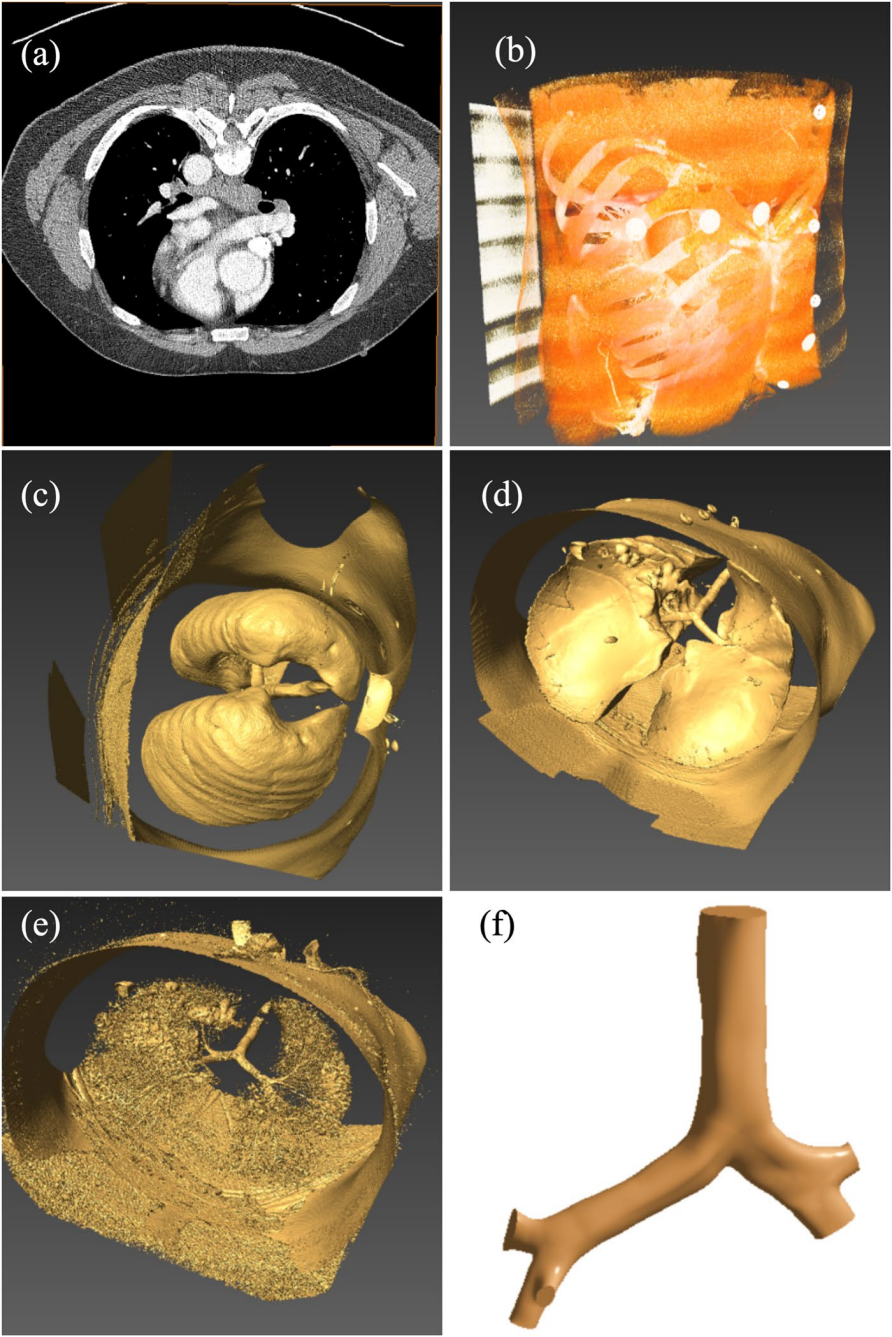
Geometry generation from the CT-based DiCom images of a healthy adult, (a) visualization of the raw DiCom images, (b) airway tree with skeleton, (c) 3D lung airway with right and left lobe, (d) bifurcation views with left and right lung, (e) constructed model with missing surface and (f) final 3-D model for the upper airways.
